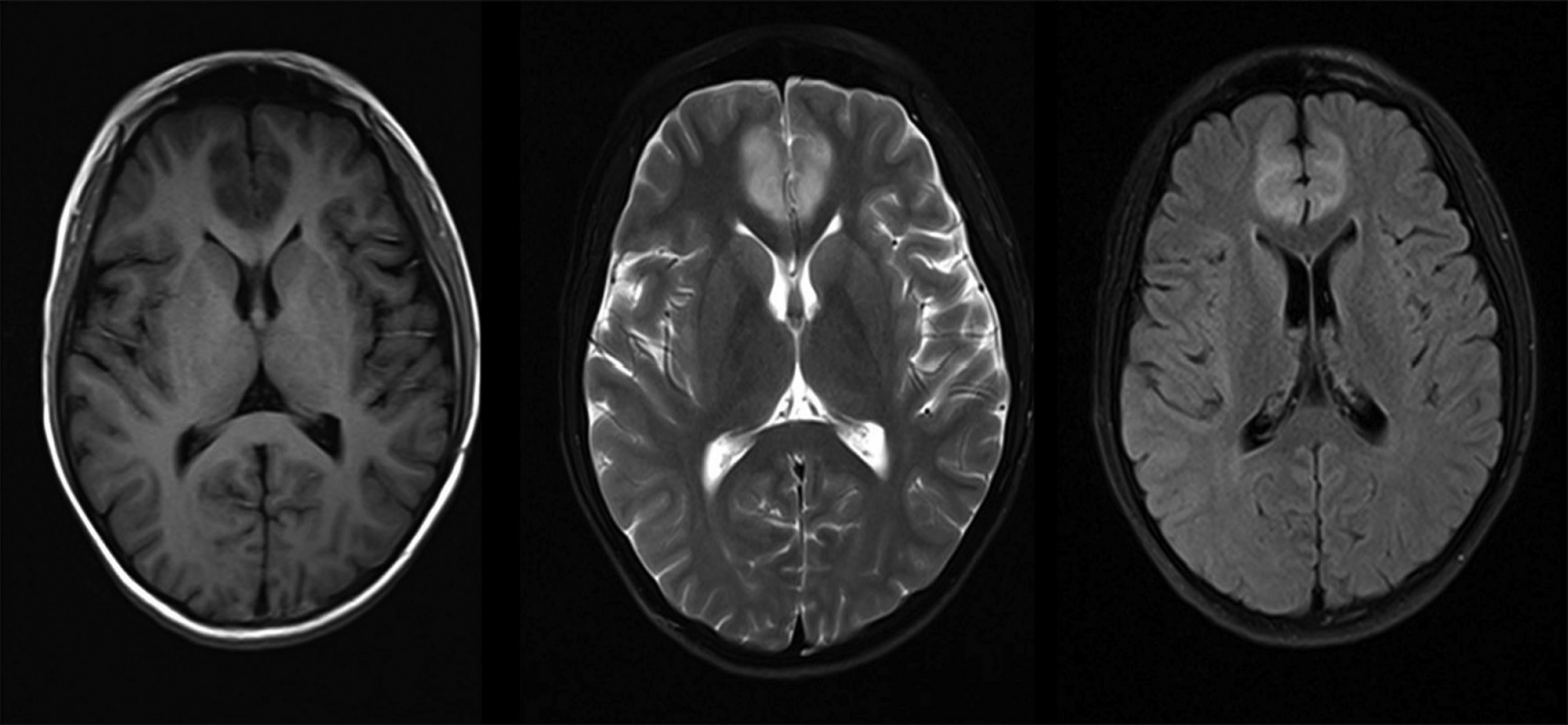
Brain MRI shows a region of high signal intensity in bilateral frontal lobe.
